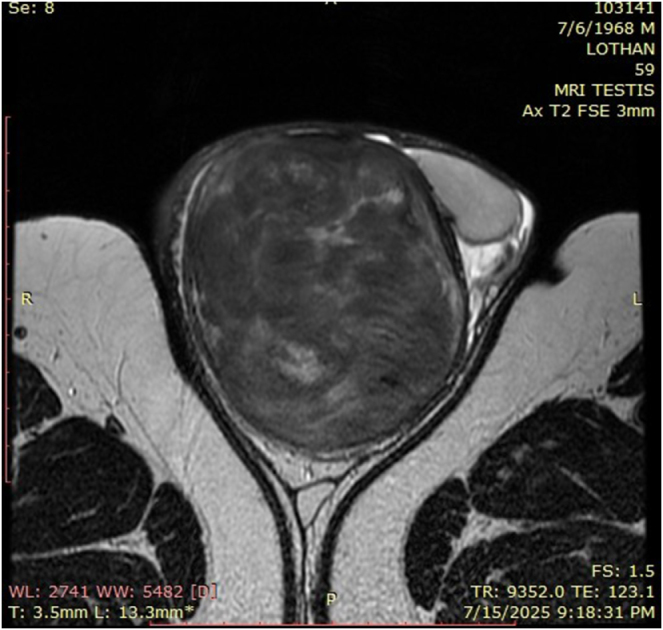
Axial T2-weighted MRI of the scrotum showing a well-circumscribed paratesticular mass adjacent to the right testis. The lesion appears as heterogeneous.
